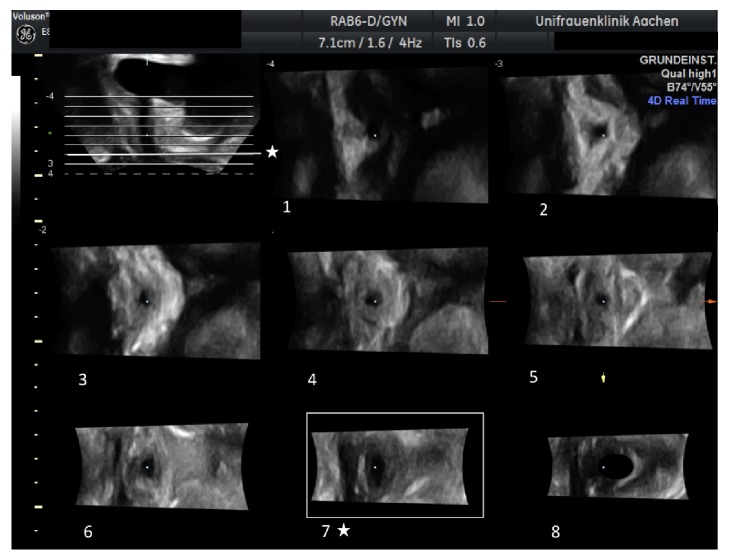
Using tomographic ultrasound imaging (TUI), 9 parallel slices are obtained (upper left figure showing the midsagittal view). The uppermost slice is placed at the meatus urethrae internus (MUI). In caudal direction eight further slices are obtained with a 4 mm interslice distance. The line marked with the symbol ☆ refers to slice number 7. This slice is shown in Subfigure 7 on the axial plane. Here, the major part of the tape is seen dorsal to the urethra.
